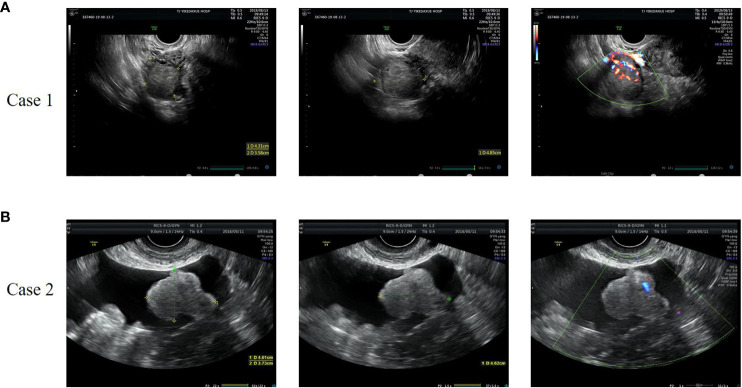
Representative images of gynecologic ultrasound of case 1 (A) and case 2 (B). (A) The ultrasound showed a 4.3×4.9×3.6cm low-moderate echo mass on the right side of the uterus with rich blood flow signals. (B) The ultrasound revealed a solid hypoechoic lobulated mass in the left adnexal area, with a size of 5.6×4.6×3.7cm and striated blood flow signal.
